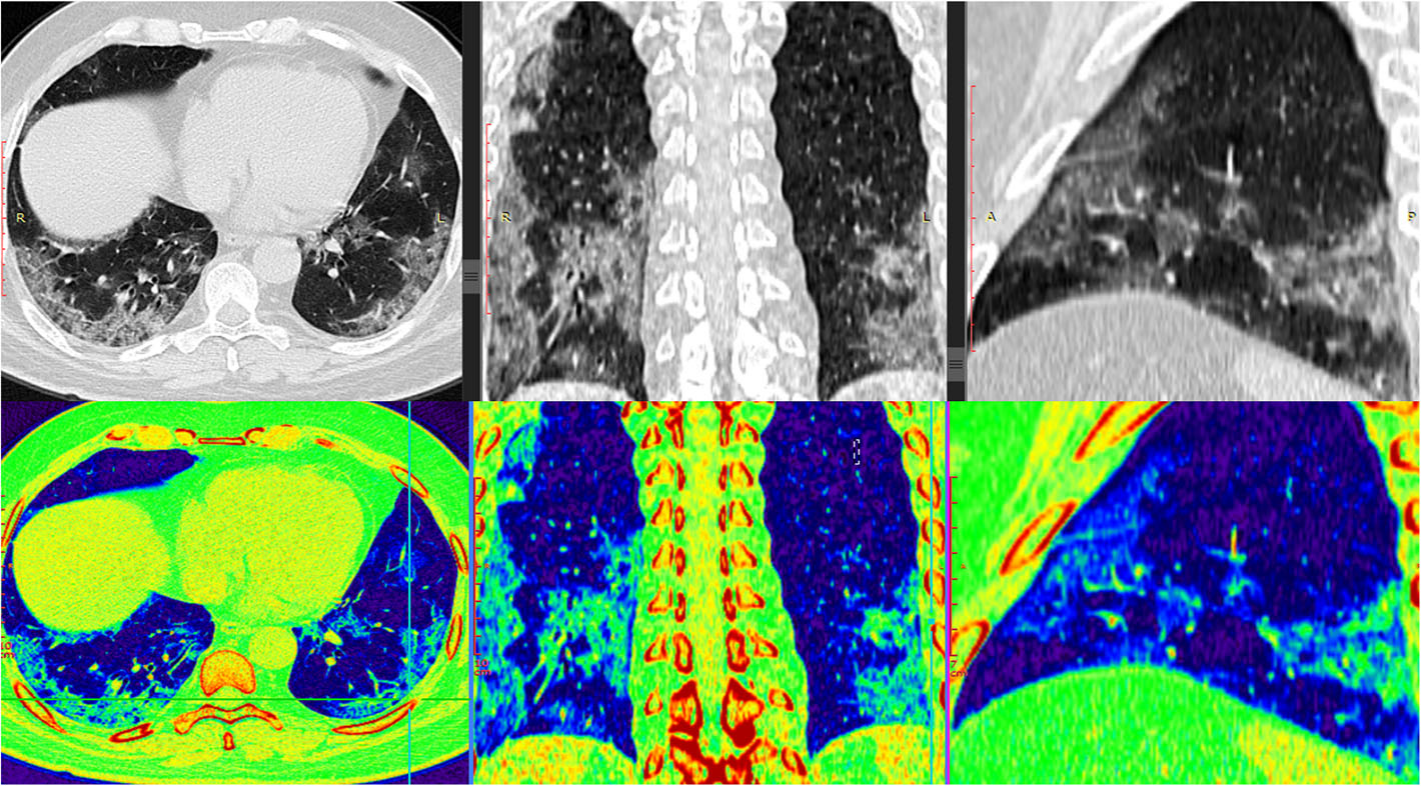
A 51-year-old male patient presented with fever, cough, and dyspnea. MSCT scan with multi-planar and color-coded images revealed widespread bilateral predominantly peripheral subpleural patchy and confluent ground glass opacities with septal and vascular thickening and reverse halo “atoll sign“. CT-SSS was 15 and serum LDH was 632. The patient exhibited severe disease course with ICU admission for 12 days followed by clinical improvement and discharge
